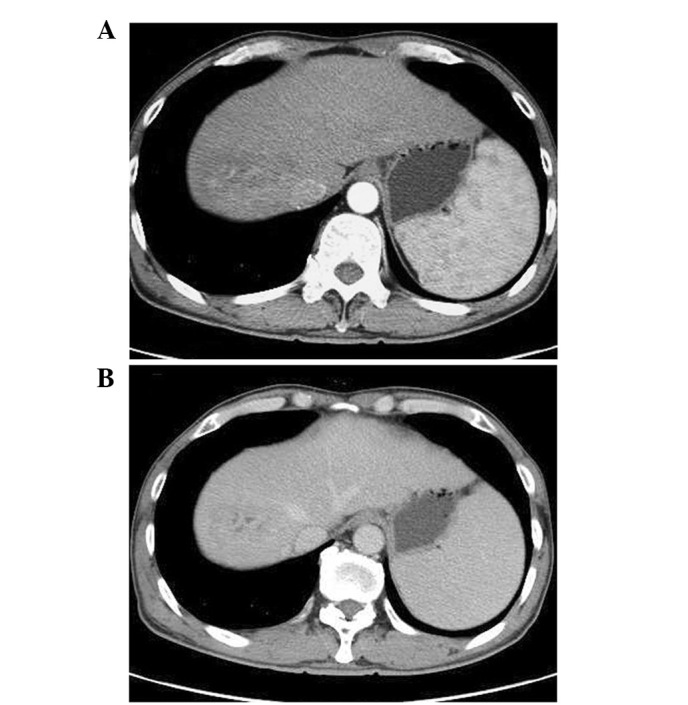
Contrast computed tomography scan demonstrated that the lesion featured a (A) mild enrichment on the arterial phase and (B) hypointense on the venous phase.
